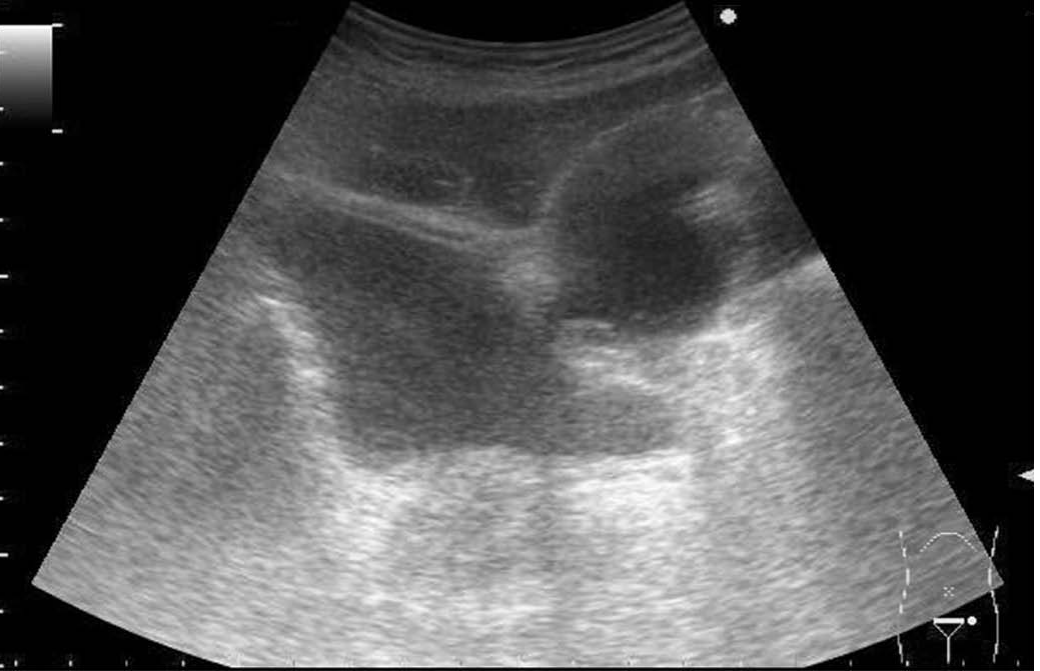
Transverse transabdominal grayscale sonogram of the pelvis showing a large bladder diverticulum.
